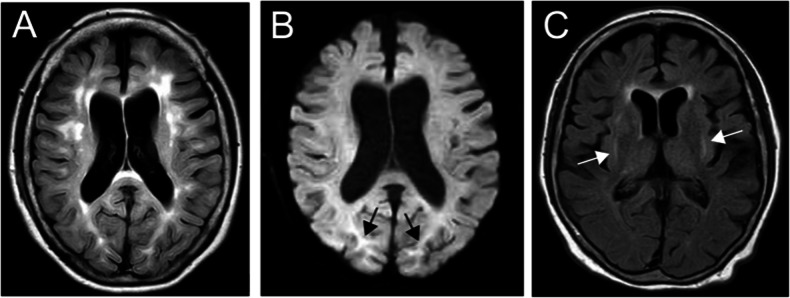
Clinical and radiological features of the patient. A FLAIR image showing periventricular hyperintensity and deep and subcortical white matter hyperintensity (axial; 1.5 T; TR, 8000 ms; TE, 100 ms). B DWI showing high-intensity signals (arrows) in the region of the corticomedullary junction in the bilateral occipital lobes (axial; 1.5 T; TR, 3732 ms; TE, 89 ms). C FLAIR image showing basal ganglia hyperintensity (axial; 1.5 T; TR, 8000 ms; TE, 100 ms).
